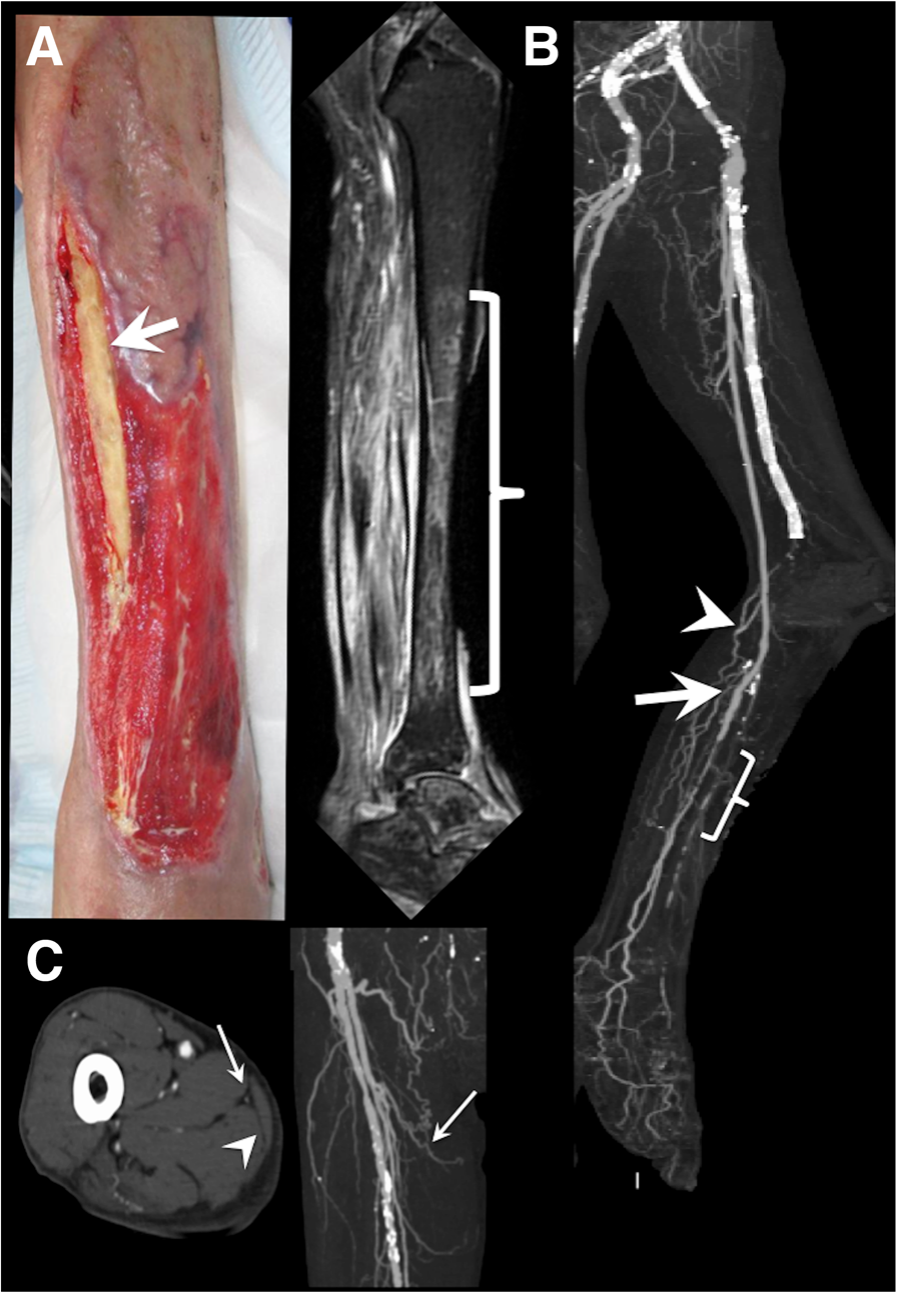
a Two months after initial surgery. A photograph showing the exposed surface of the tibia even with good granulation around the area. Magnetic resonance imaging showed osteomyelitis of the tibia (bracket). b Postoperative computed tomography showed patent bypasses to the sural artery (arrowhead) and posterior tibial artery (arrow). Native artery stenosis was detected distal to the anastomosis (bracket). c The gracilis muscle (arrowhead) and the pedicle (arrow) of the contralateral leg that originated from the medial femoral circumflex artery
